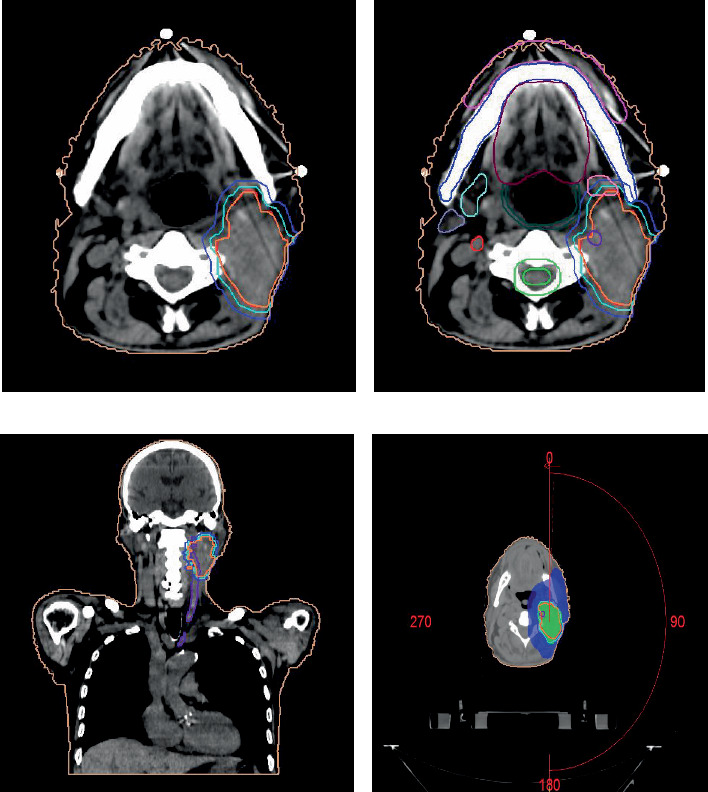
Contouring volumes on computed tomography scan for neck node. (a) Axial section: GTV node in bright red: 66 Gy, CTV in cyan: 59.4 Gy, PTV in purple: 59.4 Gy. (b) Axial section: lips: pink, mandible: dark blue, right parotid: mauve, right submandibular gland: light blue, oral cavity: maroon, right carotid artery: red, spinal cord: green, spinal cord planning risk volume (PRV): light green, left submandibular gland: light pink, left carotid artery: dark purple. (c) Coronal section showing GTV encasing left carotid artery. (d) Axial section showing dose coverage of the GTV.
